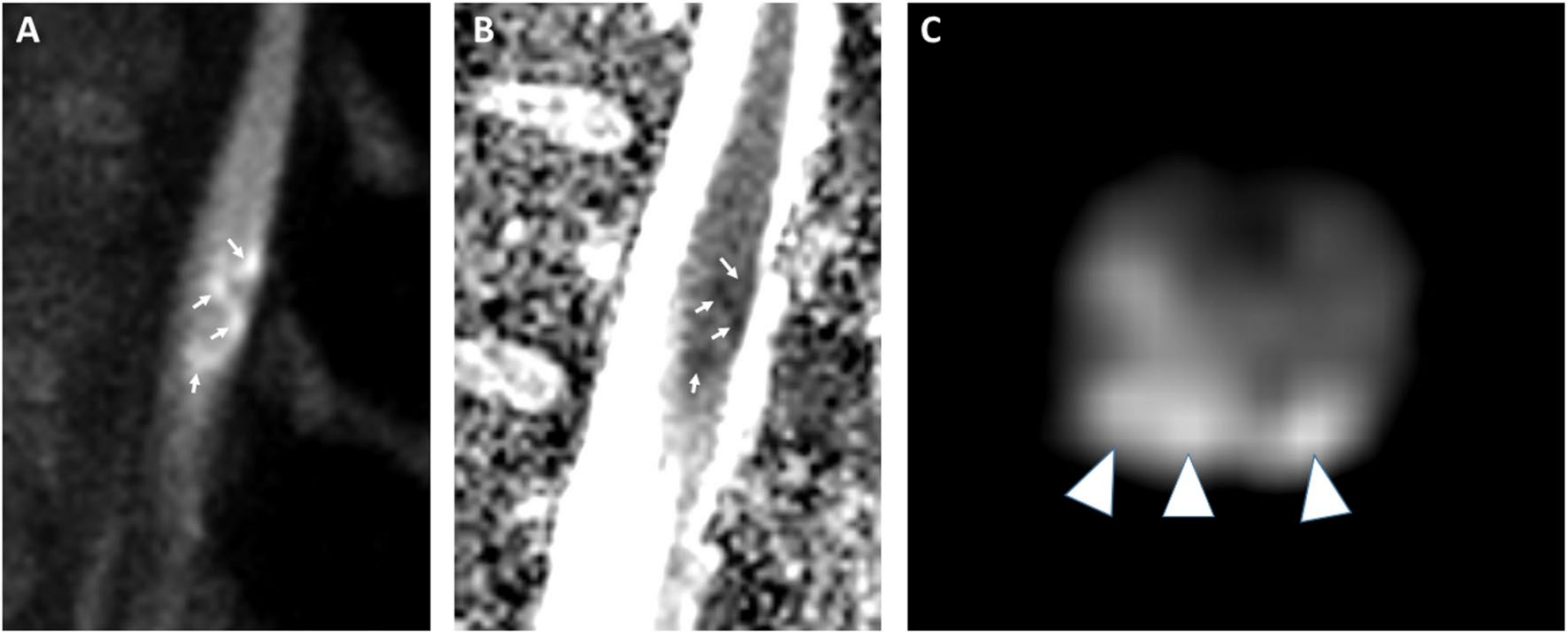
Sagittal diffusion weighted imaging (DWI) shows diffusion restrictions (arrows in A, b600; arrows in B, apparent diffusion coefficient) matching the T2-hyperintensities and consistent with acute conus medullaris infarction. Transversal DWI confirms the predominantly posterior involvement (arrowheads in C, b600)
